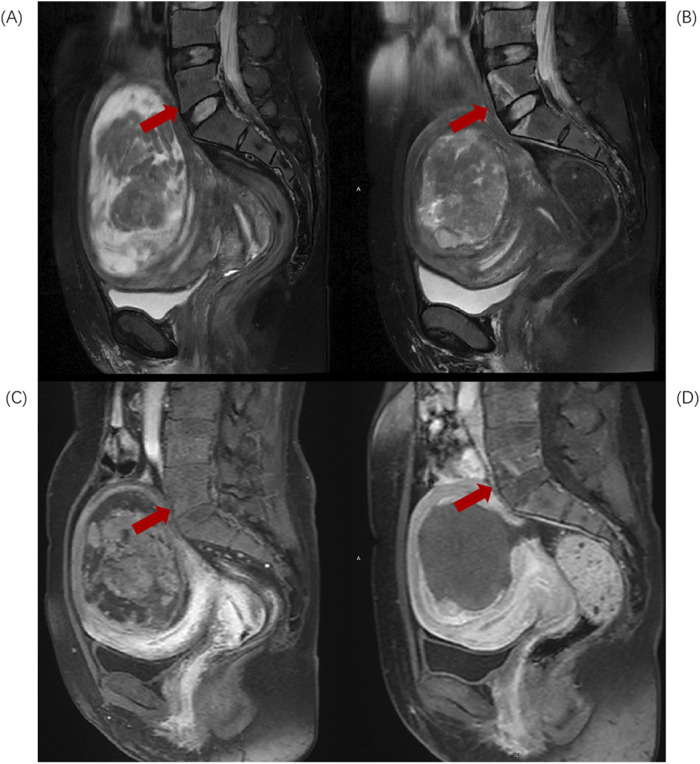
Sagittal MR images of a 43-year-old patient with uterine fibroids before and after HIFU ablation. (A) Pre-HIFU T2WI image shows normal signal intensity in L5 and S1 (arrows); (B) Post-HIFU T2WI shows patchy mixed high and low signal areas in L5 and S1 (arrows); (C) Pre-HIFU enhanced imaging shows normal enhancement in L5 and S1 (arrows); (D) Post-HIFU enhanced image shows decreased perfusion in the mixed high and low signal area of L5 and L1 on T2WI (arrow).
